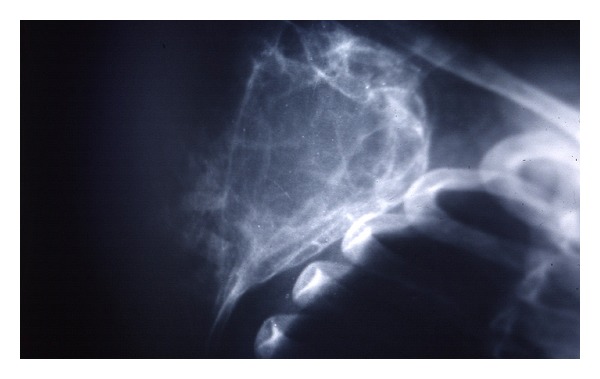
Preoperative scapular view radiograph of the right scapula.
